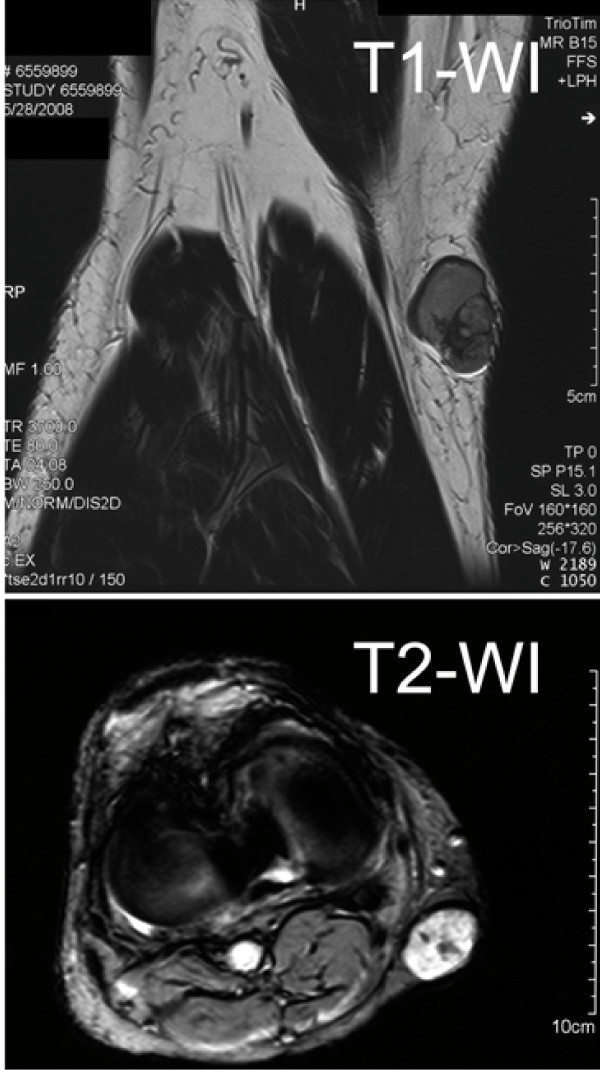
MRI showing a mass lesion embedded in the subcutaneous tissue on the medial posterior side of the right knee, demonstrating low and high signal intensity in T1- and T2-weighted images (arrows in upper and lower), respectively.
